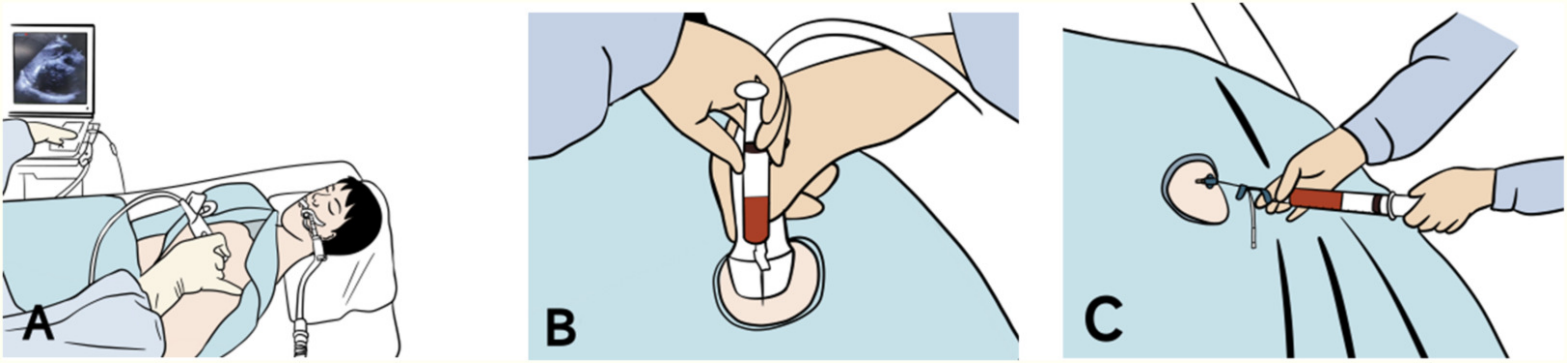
(A) Emergency bedside ultrasound showing localization of pericardial effusion. (B) First aspiration yields approximately 4 mL of pericardial effusion after needle puncture. (C) Second aspiration yields approximately 6 mL of pericardial effusion after successful placement of the drainage catheter.
